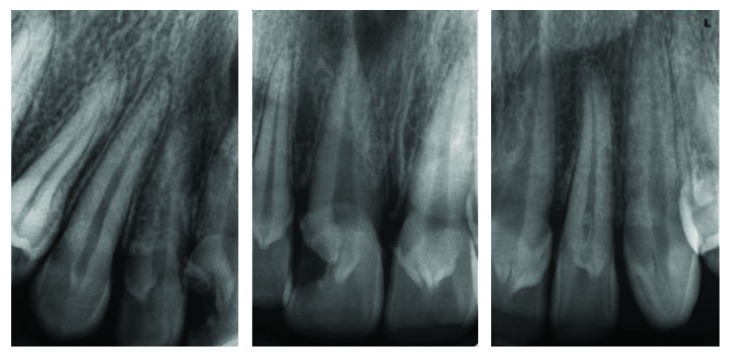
Case 2: periapical radiograph shows radiographic appearance of talon cusp.
